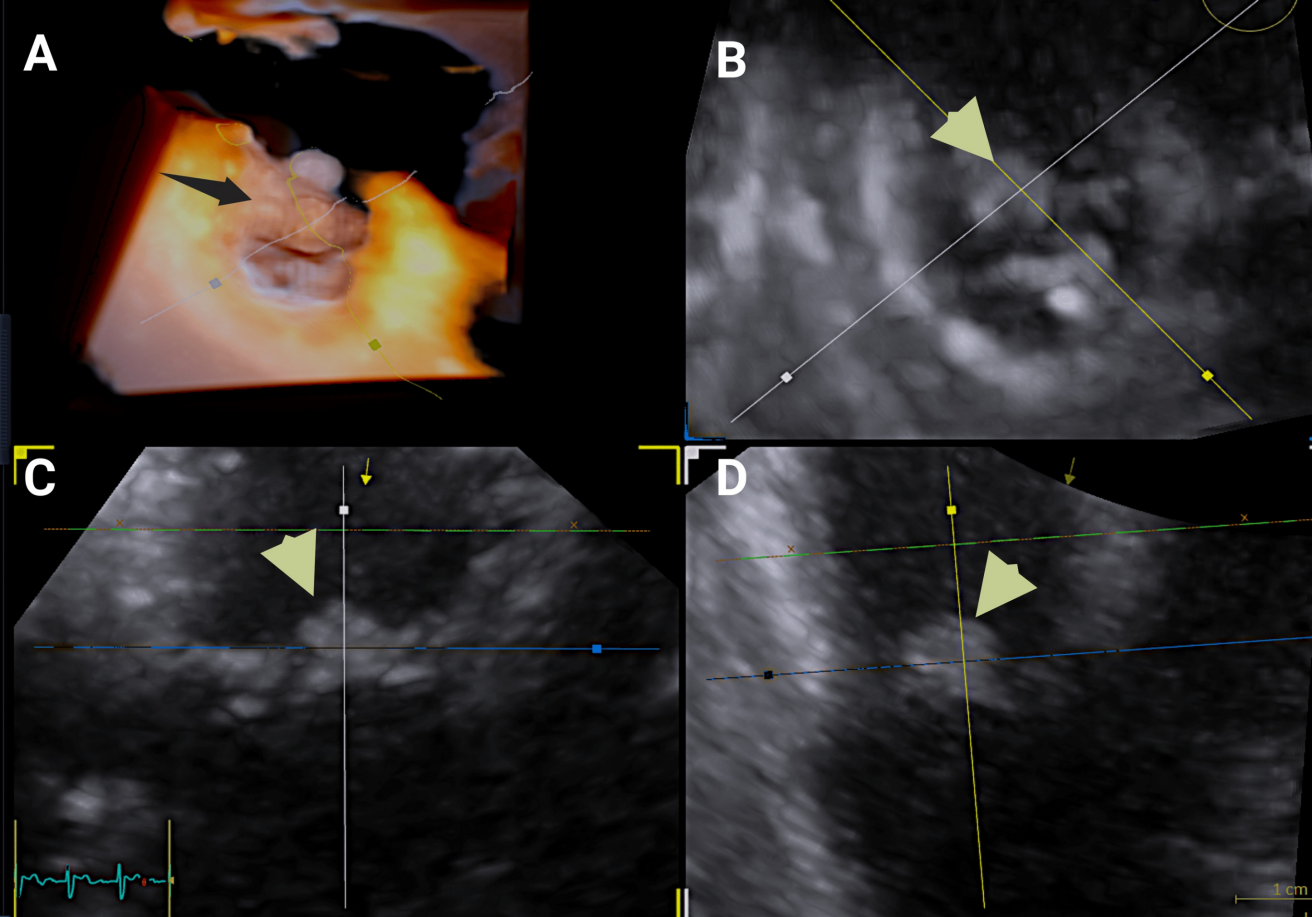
Flexislice transillumination 2D and 3D transesophageal echocardiography images. Flexislice transillumination 2D and 3D transesophageal echocardiography of the pulmonary valve (panel A). A vegetation was identified attached to the anterior cusp of the pulmonary valve (black and yellow/green arrows) (panels B-D).
